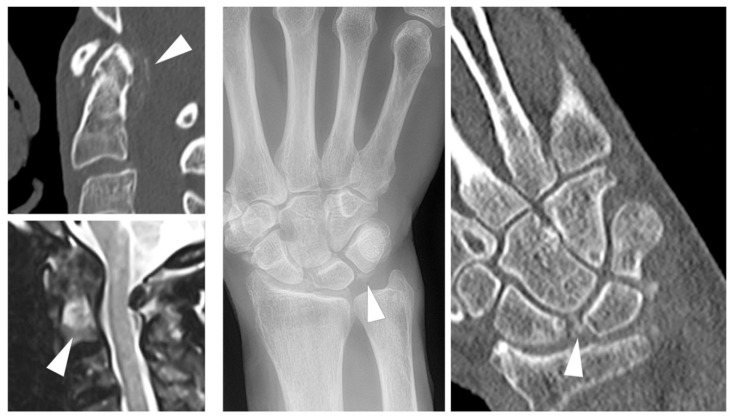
Multimodality imaging in Calcium Pyrophosphate Dihydrate Deposition (CPPD). (Left): Crowned dens syndrome with flake-like calcifications in the CT image (arrowhead) and concurring bone marrow edema on MRI (arrowhead). (Right): CPPD of the wrist, showing calcifications of the luno-triquetral ligament on radiography and additional calcifications of the scapho-lunate ligament on CT (arrowhead).
